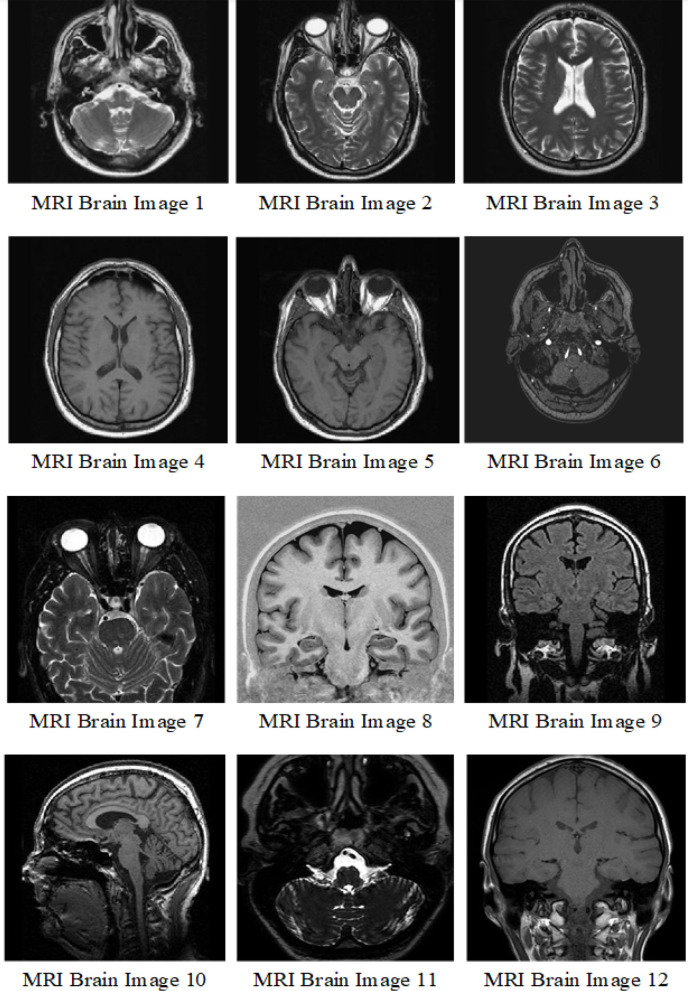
Original MRI brain images.
